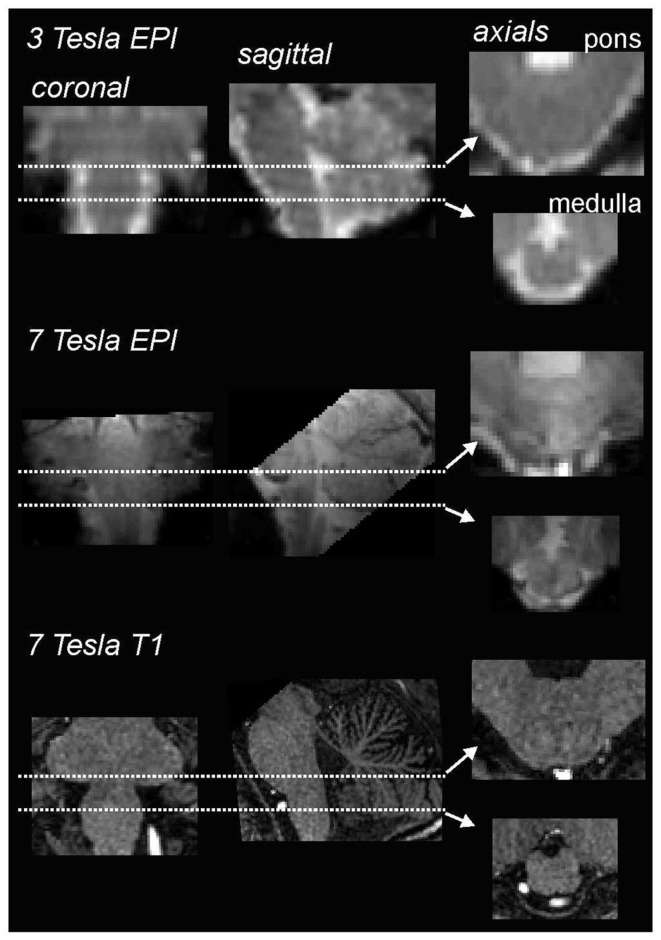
Comparison of coronal, sagittal, and axial echo-planar images (EPI) through the medulla collected using a 3 T (top row) and 7 T (middle row) MRI scanner collected in the same individual. Note that the images collected using the 7 T scanner have finer spatial resolution and greater gray/white differentiation. The bottom row shows a series of T1-weighted anatomical images also collected using a 7 T MRI scanner.
